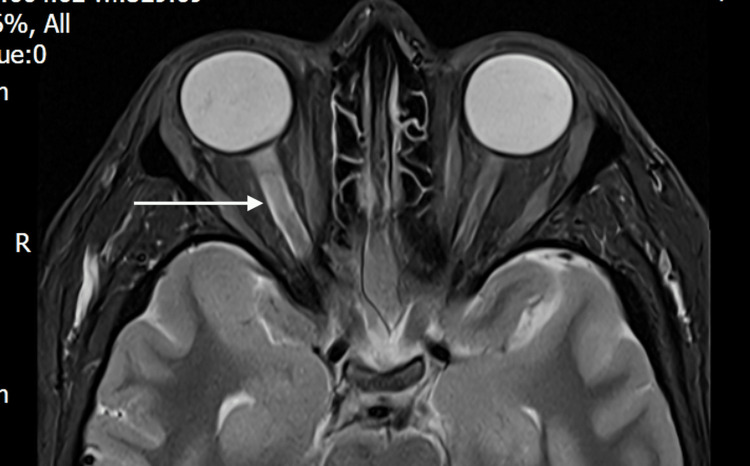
Axial T2-weighted fat-suppressed MRI of the orbits, performed shortly after symptom onset, showing hyperintensity and swelling of the right optic nerve (arrow) consistent with acute optic neuritis, with no additional intracranial abnormalities
